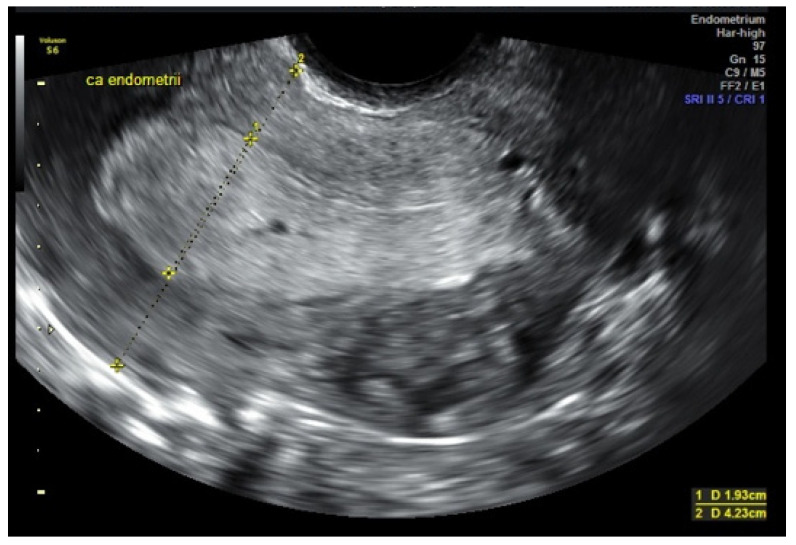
Transvaginal sonography (TVS imaging). Longitudinal section. Endometrial cancer, stage IA, G1, infiltration with homogeneous echogenicity.
